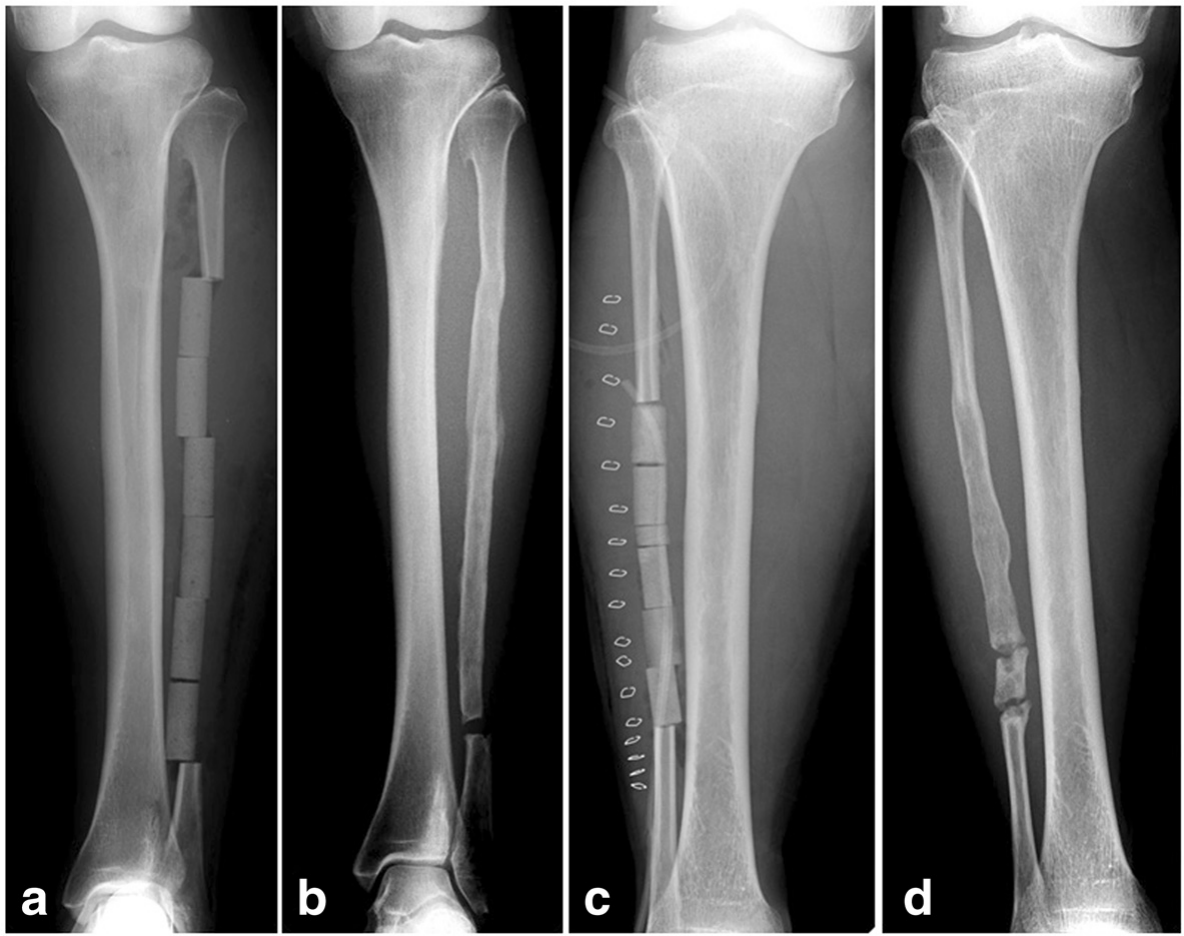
Donor sites of fibular strut. (a) Patient 4. β -TCPs were implanted at the donor site of fibula. (b) Eight years after surgery, the implanted β -TCPs were replaced by newly formed bone. (c) Patient 5, a 69-year-old male. A-P X-rays soon after surgery (c) and three years after surgery (d) indicated regenerated new bone formation
